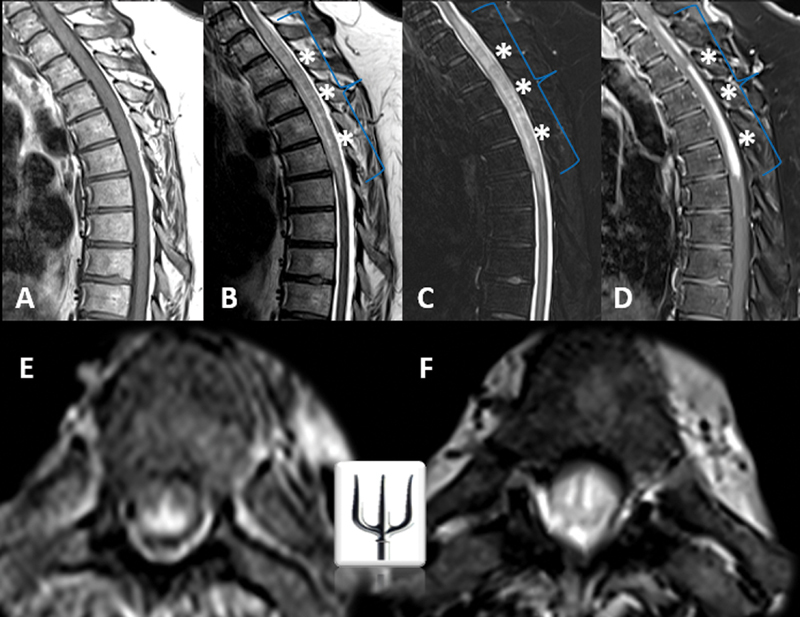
Magnetic resonance imaging of the thoracic spine in sagittal (
